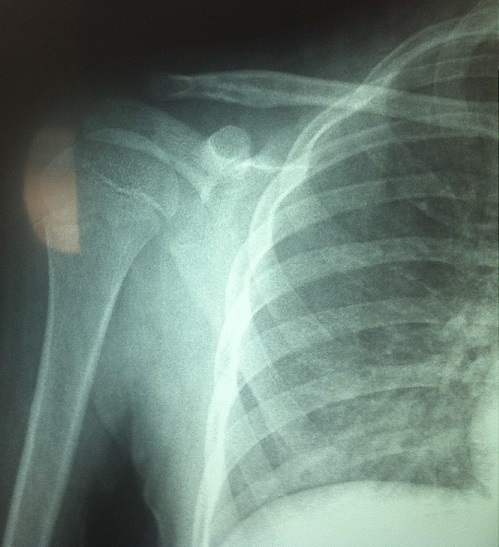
Radiographie postopératoire après un an montrant l’étendue de la résection avec un bon remodelage osseux
